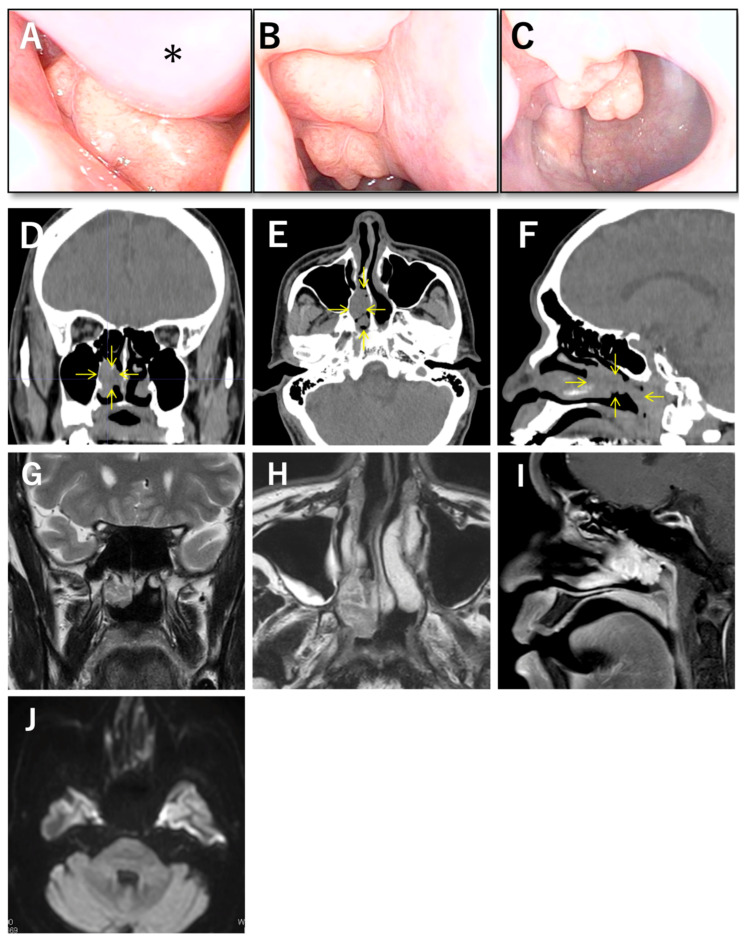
(A) Endoscopic view showing the anterior surface of the right nasal cavity mass. The asterisk (*) indicates the right middle turbinate. (B) The mass appears milky-white, smooth-surfaced, and lobulated. (C) The posterior end of the mass extends to the choana. (D–F) Coronal, axial, and sagittal non-contrast CT images of the paranasal sinuses showing a soft-tissue-density lesion measuring approximately 28 mm in the posterior right nasal cavity, without evidence of adjacent bone destruction or localized sclerosis. (G,H) Coronal, axial, and sagittal MRI findings. On T2-weighted images, the lesion showed a heterogeneous low-signal-intensity area. (I) On contrast-enhanced T1-weighted images, faint enhancement was observed mainly along the lobulated surface of the mass; however, there was no marked difference compared with the surrounding nasal mucosa of the septum and middle turbinate. (J) Diffusion-weighted imaging revealed no restricted diffusion within the lesion.
